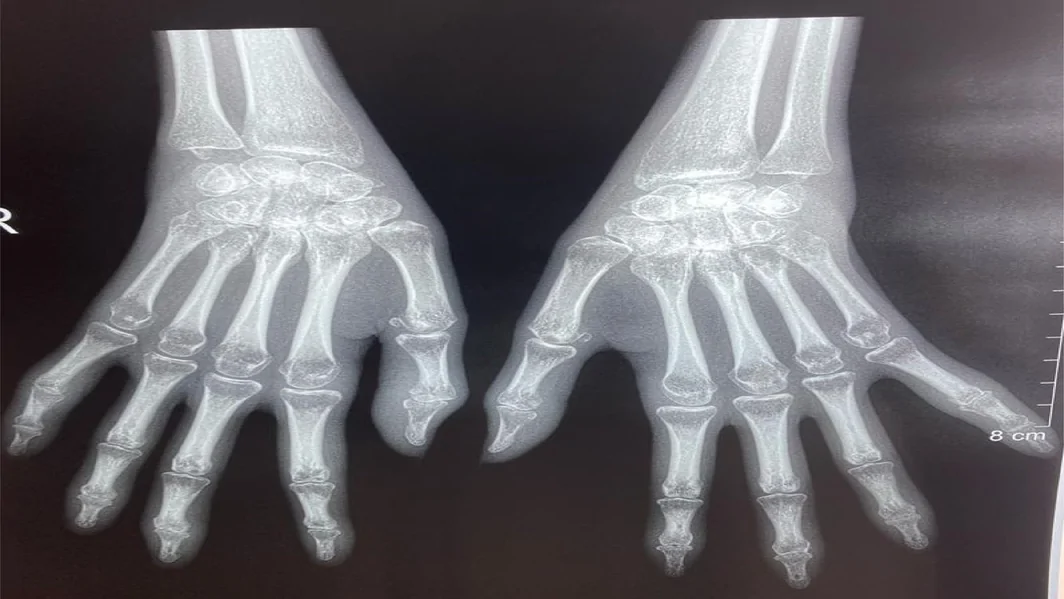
Hand radiograph showing diffuse demineralization and soft tissue thickening around the distal interphalangeal joints.
